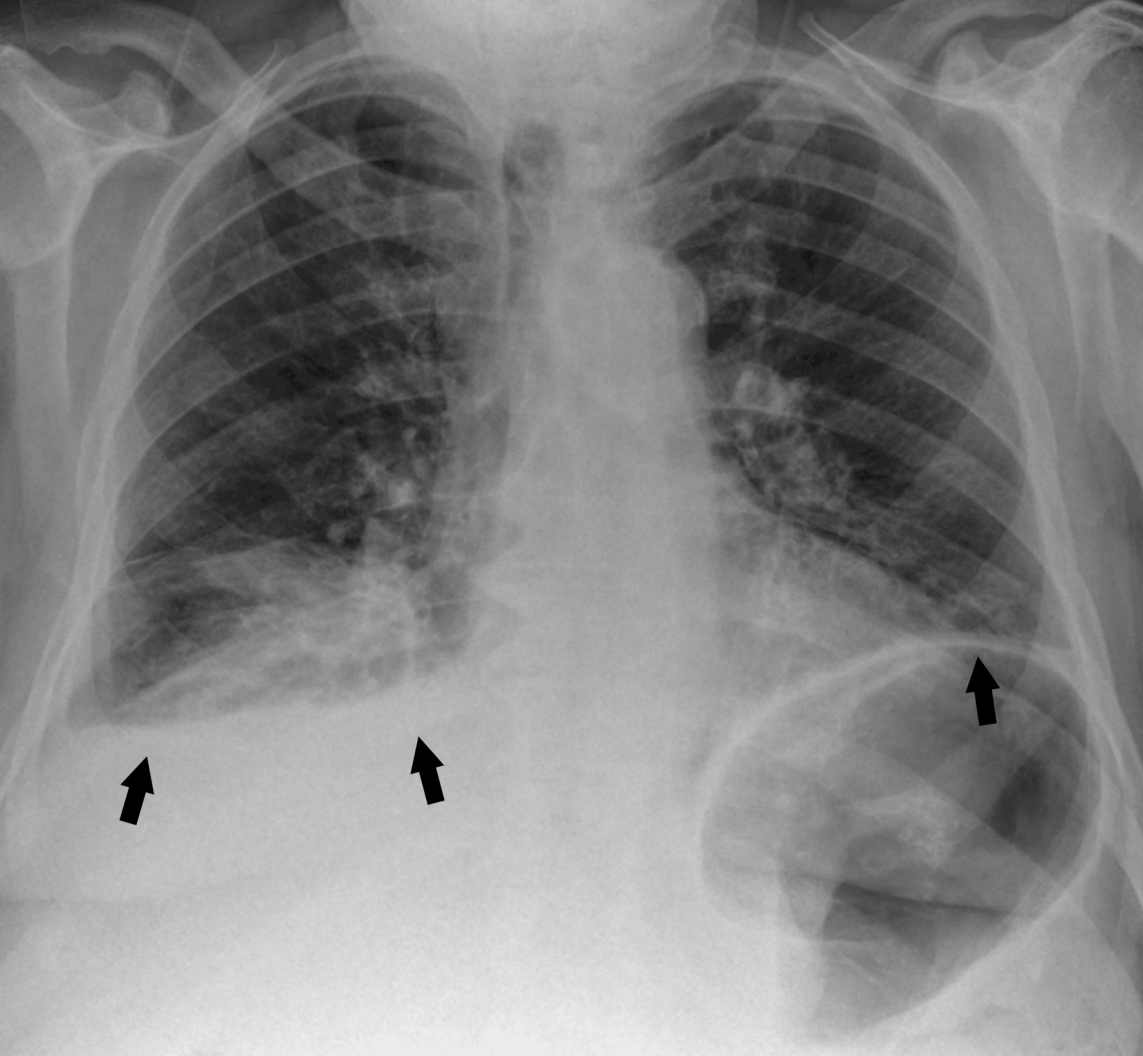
Patient's chest X-ray upon Emergency Department admission showing bilateral infiltrates (arrows), predominantly on the right side, consistent with pneumonia.
